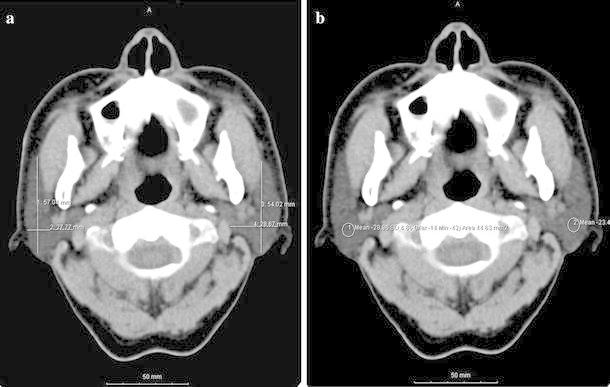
a Method for determining the sizes of the parotid glands. Cross-sectional long/short diameters, showing the maximum area of the parotid glands, were measured on axial plain CT images. b Method for determining the CT numbers of the parotid glands. *Circular regions* of interest were selected on the posterolateral regions of the parotid glands, avoiding the retro-mandibular veins
